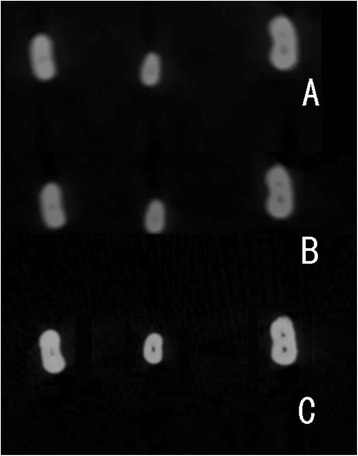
Example of CBCT images of the same incisor near to the apical region, (a) was in FS mode, (b) was in ZS mode and (c) was in HZ mode. The root canal morphology in HZ mode is much sharper than the other modes
